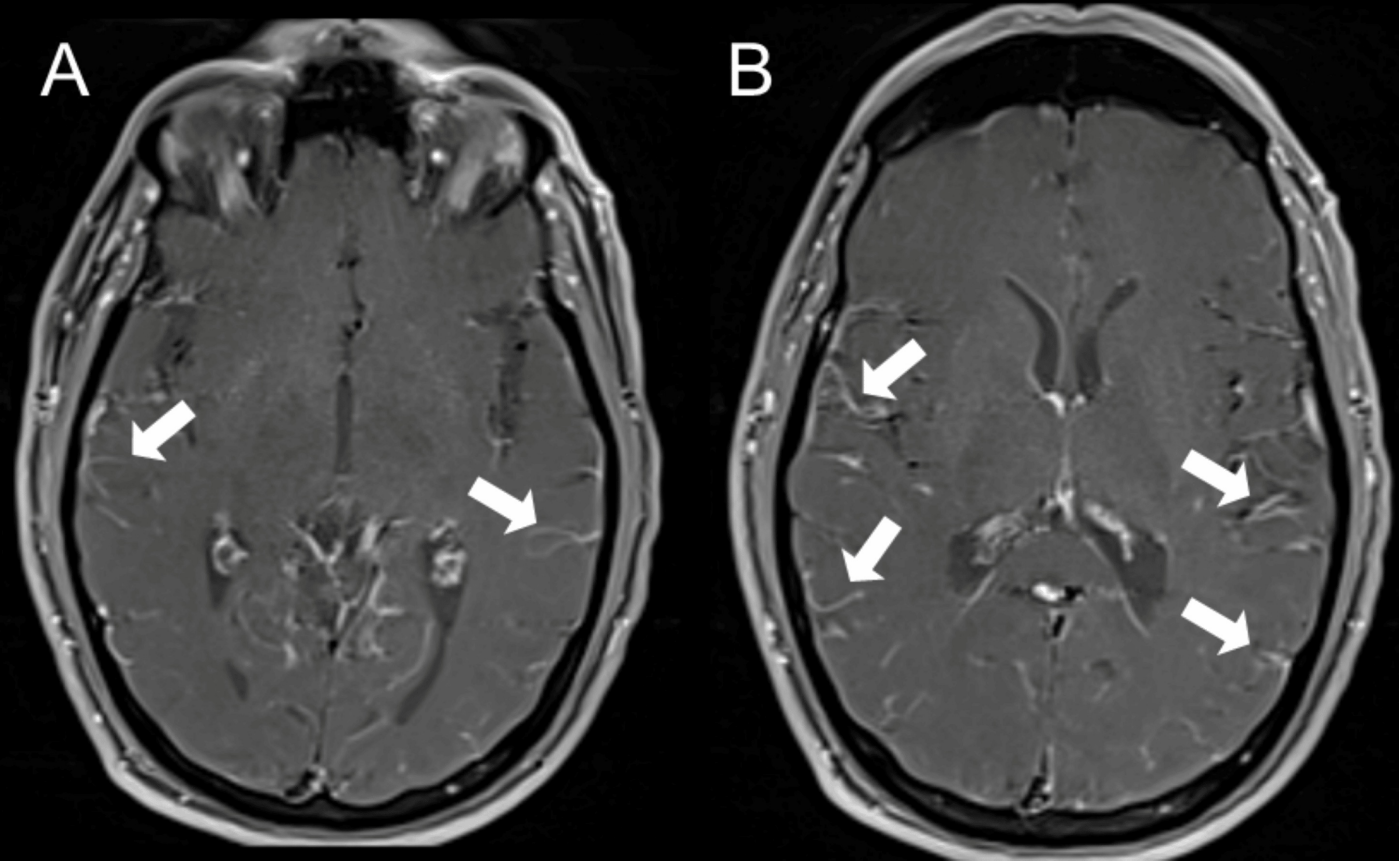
Contrast-enhanced axial T1-weighted sequence shows diffuse leptomeningeal enhancement (A and B). The enhancement is both pia-arachnoid, which extends into the subarachnoid spaces of the sulci (arrow).
